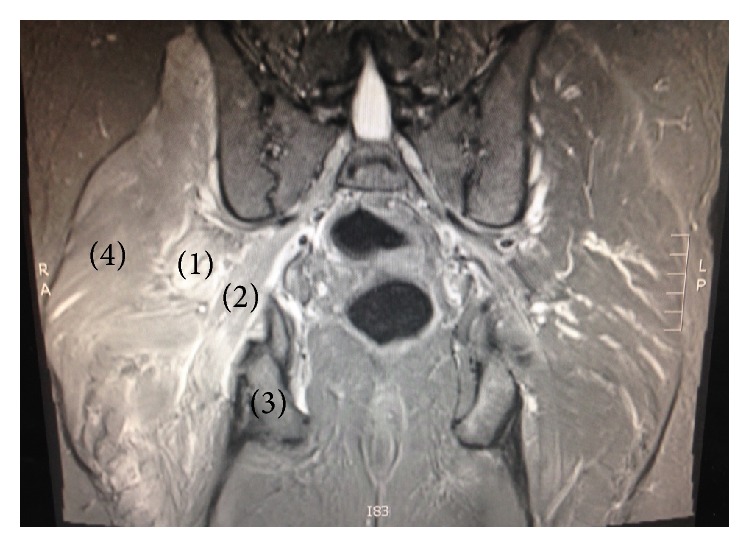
Signal abnormality of the right short external rotators on coronal section of T2-weighted MRI. (1) Piriformis. (2) Obturator internus. (3) Ischium. (4) Gluteus maximus.
